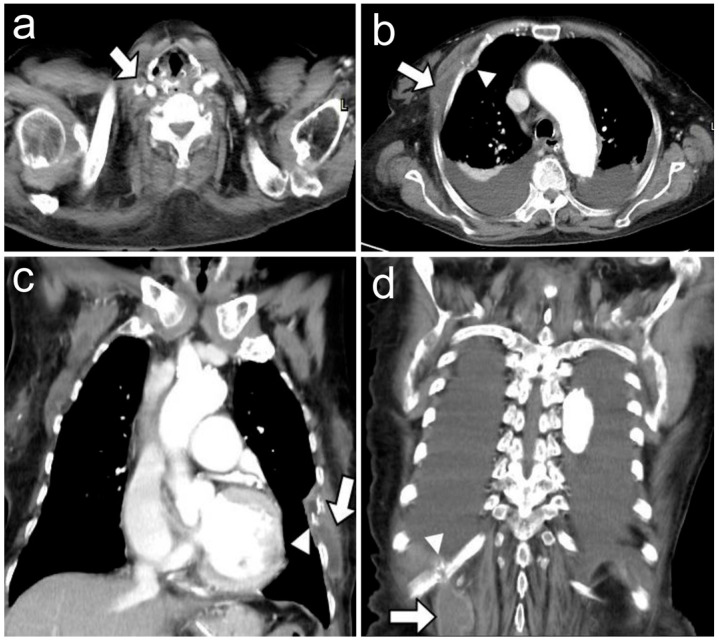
Contrast-enhanced computed tomography of the chest shows a filling defect in the right internal jugular vein (arrow) (a) and three low-density lesions with rim enhancement in right pectoralis major muscle (arrow) with destruction of right anterior third rib (arrowhead) (b), left chest wall (arrow) with destruction of left sixth rib (arrowhead) (c), and right posterior chest wall (arrow) with destruction of right eleventh rib (arrowhead) (d).
